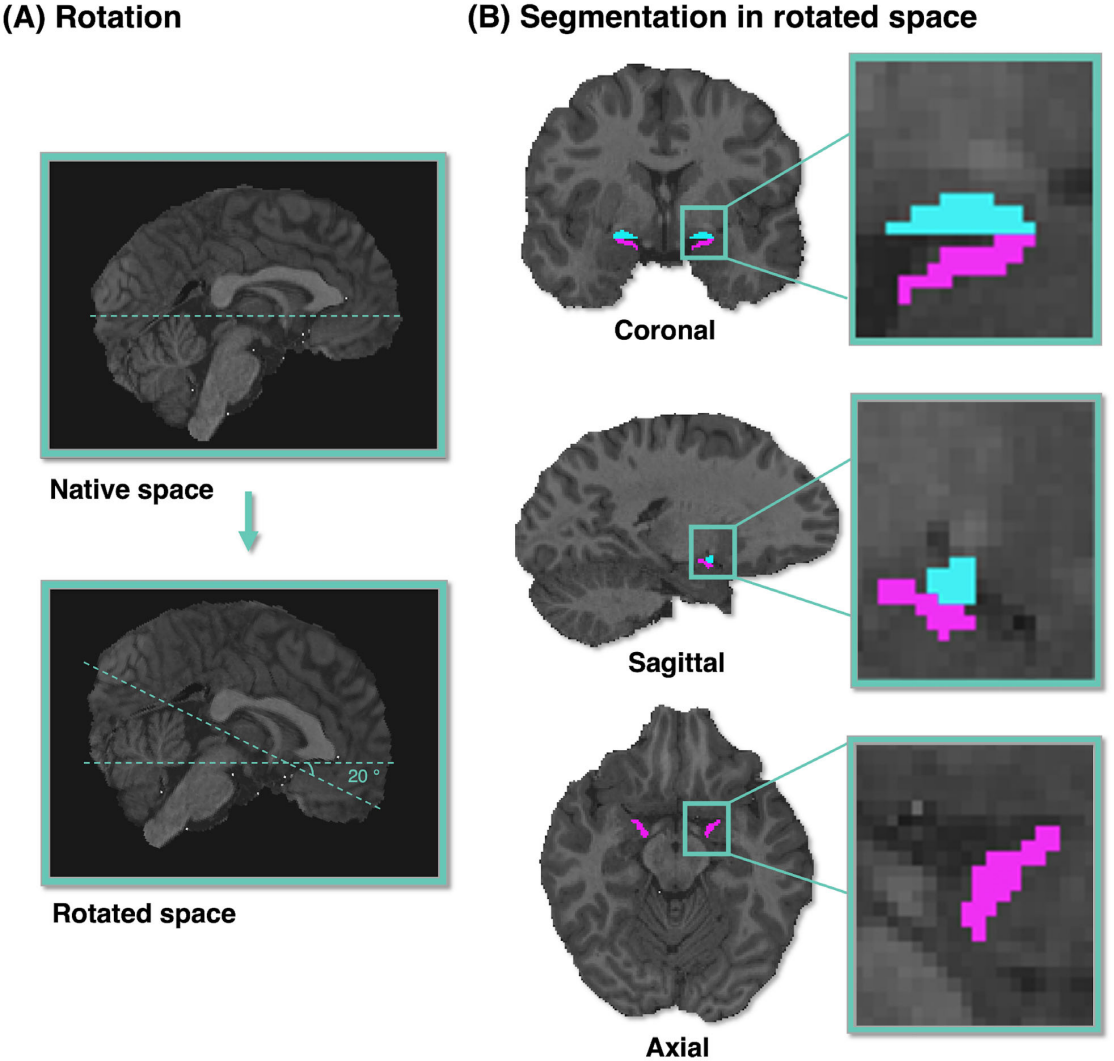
(A) Rotation of the nonenhanced T1‐weighted MRI relative to the anterior commissure by +20°. (B) Sagittal, coronal, and axial view of the piriform masks of a representative subject with epilepsy on a structural T1‐weighted MRI. Color scheme: Blue = frontal PIC; pink = temporal PIC.
